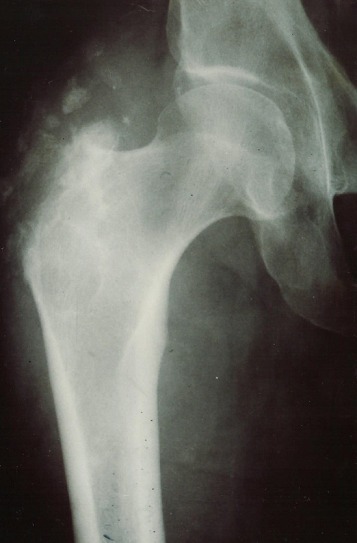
Image radiographique des remaniements du grand trochanter
